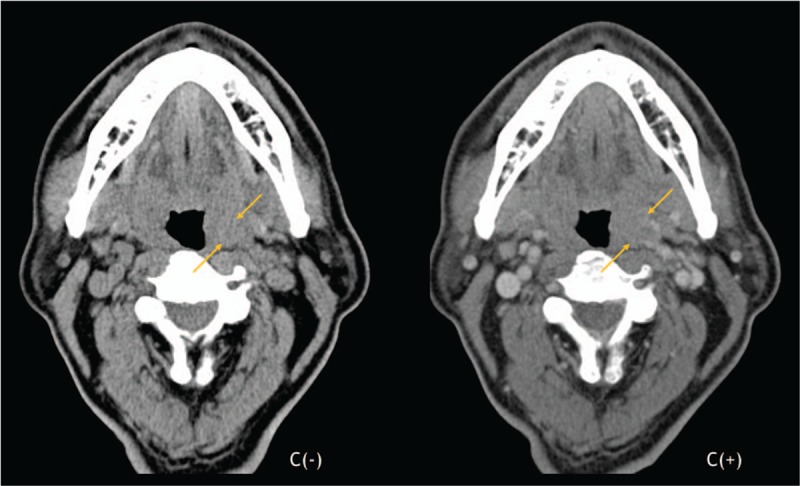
Neck computed tomography (CT) scan revealed a 29 × 20 mm homogenous lesion with enhancing soft tissue in the left palatine tonsil (arrow), without suspicious lymph node metastasis.
